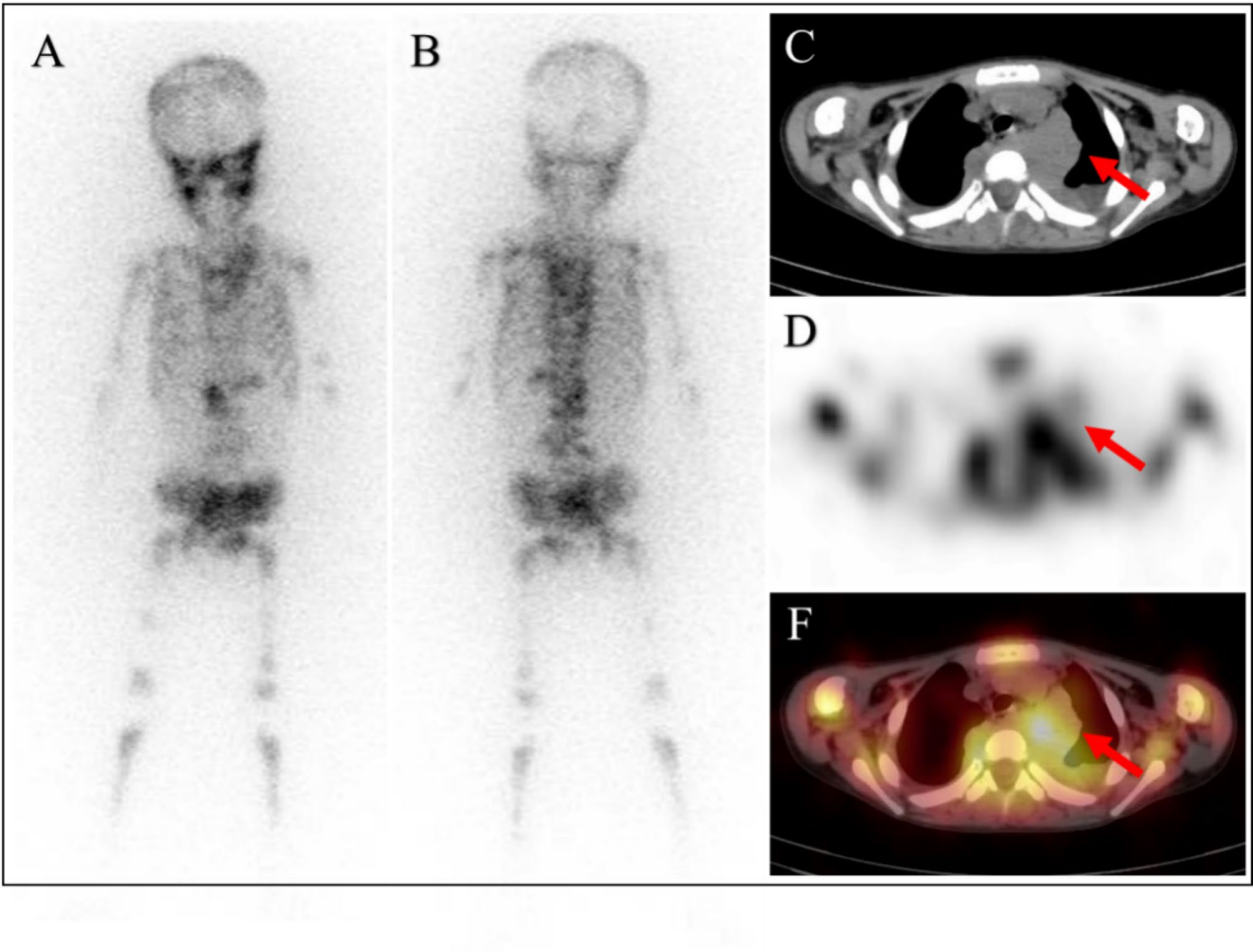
A four years old boy was diagnosed as having left-chest paraspinal neuroblastoma. The 123I-MIBG whole-body and axial SPECT/CT images revealed intense tumor activity (A, anterior, B, posterior, C, CT, D, SPECT, E, fusion). The IDRFs counts were 7, with a maximum tumor diameter of 5.1. The Curie score measured 28, and there were indications of metastasis in the whole-body bone/bone marrow. A ratio of 10 was observed in Tmax/Lmax, while ASP reached 150%. Unfortunately, the patient died after 184 days
